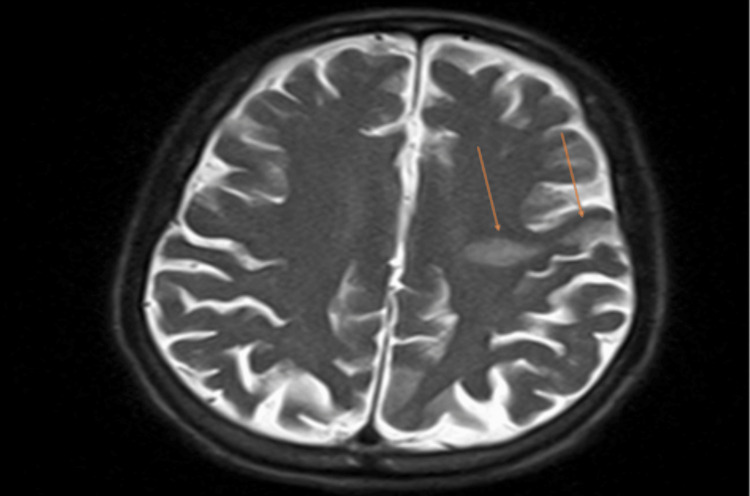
MRI brain without contrast in the T2/FLAIR sequence showing a hyperintense lesion consistent with an infarct in the left precentral gyrus, as indicated by the orange arrows. MRI, magnetic resonance imaging
